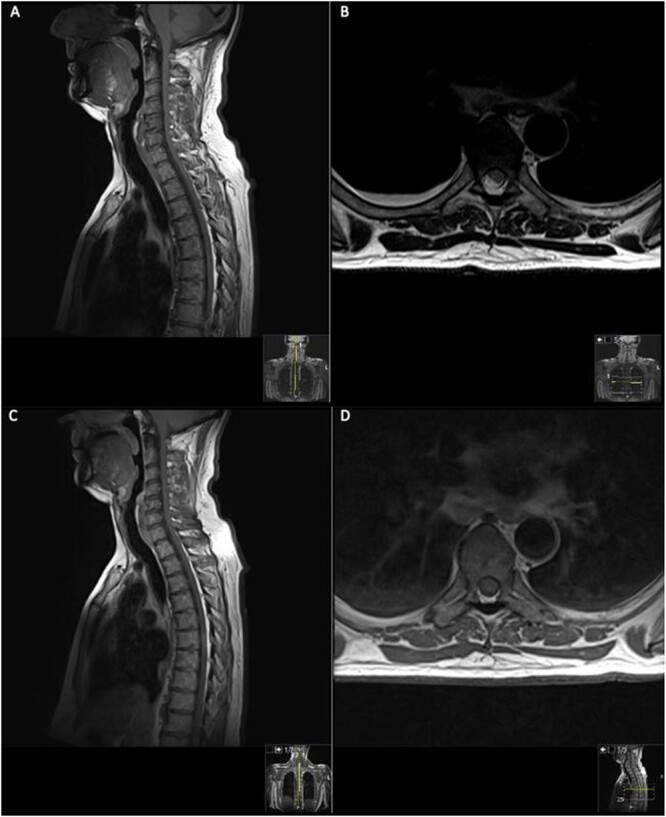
MRI on initial presentation; T1 (A) sagittal view sowing mild high signal changes alongside a T2 sequence transverse view (B) which shows the intermediate signal change; when compared with post-contrast T1 sagittal and transverse imaging (C) and (D), showing there is no significant enhancement post-contrast. MRI revealed an intramedullary spinal cord lesion at the level of T6 measuring 24 mm in craniocaudal dimension.
